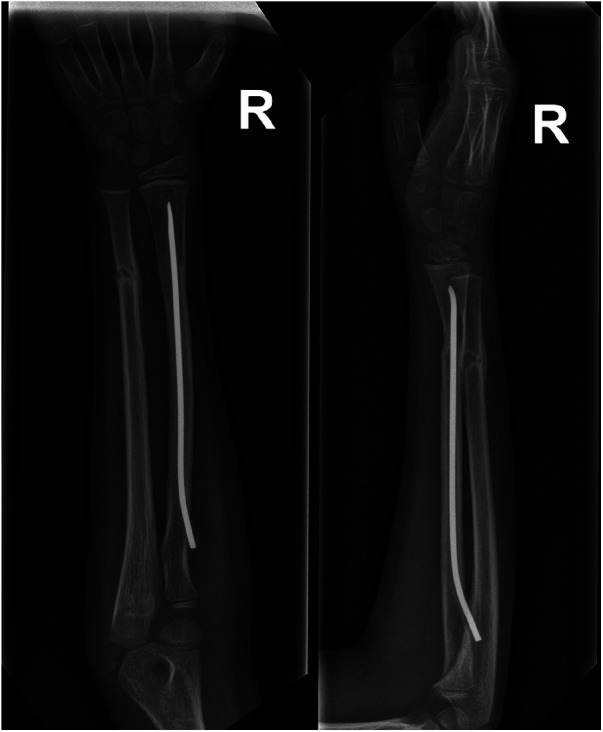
Seven weeks after initial surgery, a follow-up x-ray showed progressive bone union with proper bone alignment. In the radius, a flexible intramedullary nail inserted from the proximal metaphysis.
